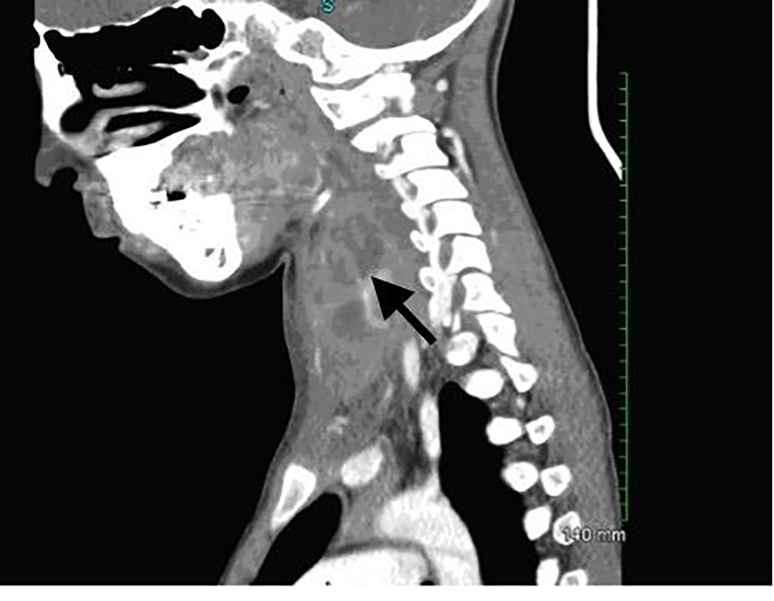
Saggital computed tomography of the neck demonstrating multiloculated heterogeneous fluid (arrow) in the left thyroid lobe.
